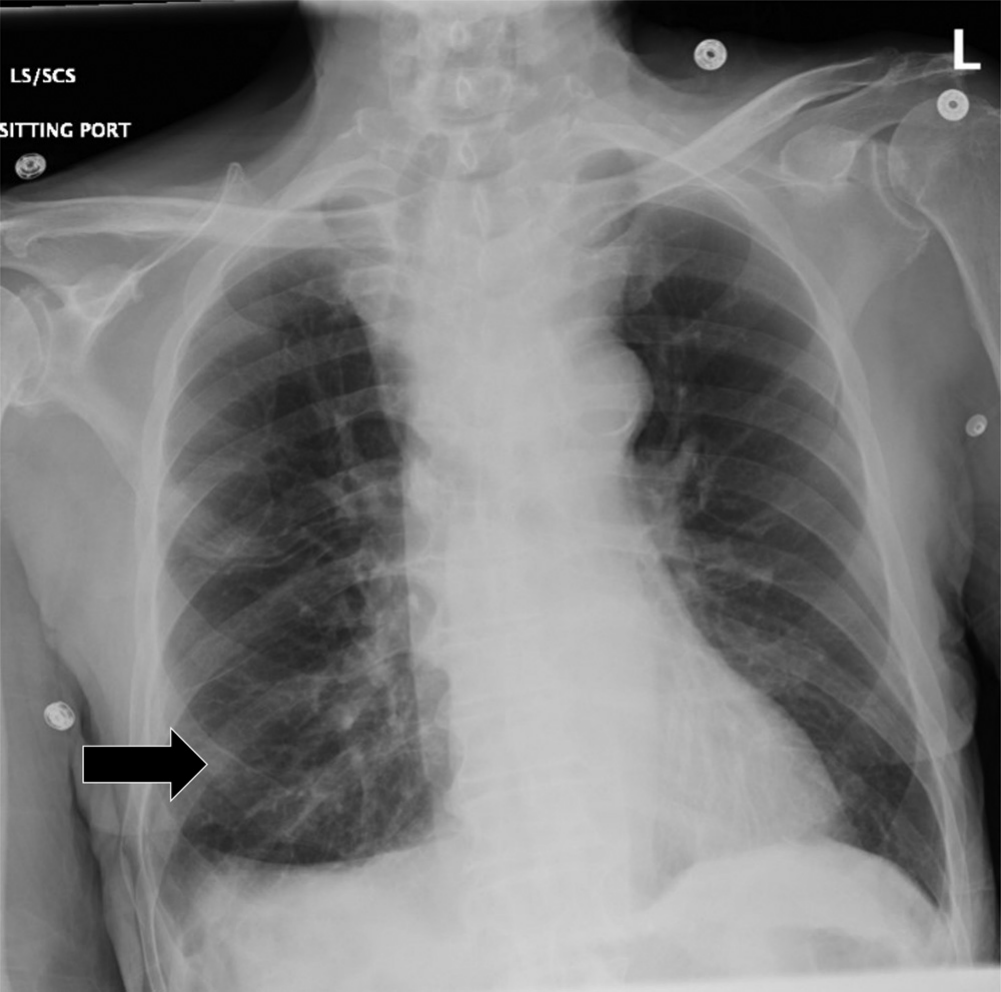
Initial chest radiograph showing an opacity in the right midlung (black arrow) in a 77-year-old man before he experienced a rapid change in mental status.
